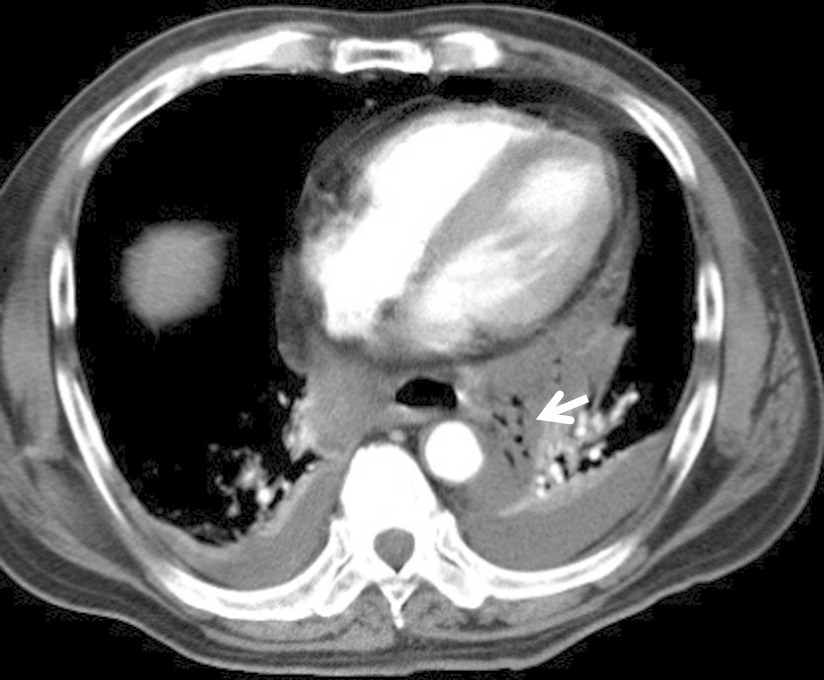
CT findings. Pneumomediastinum is shown indicating mediastinitis (arrow)
